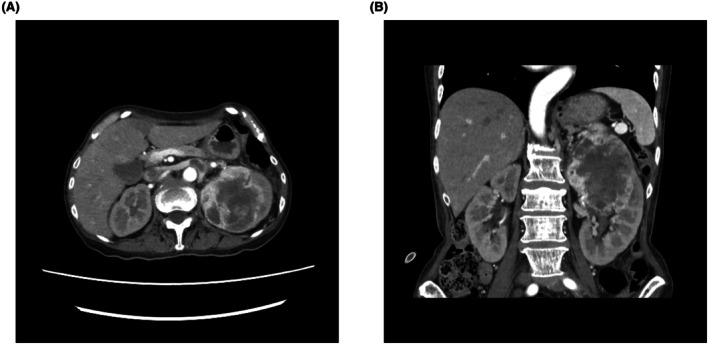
Abdominal computed tomography (CT) scans showing the left renal mass. (A) Axial view of a contrast‐enhanced CT scan showing a large, well‐defined mass (approximately 8 cm in diameter) in the left kidney. (B) Coronal view of the CT scan showing the left renal mass.
